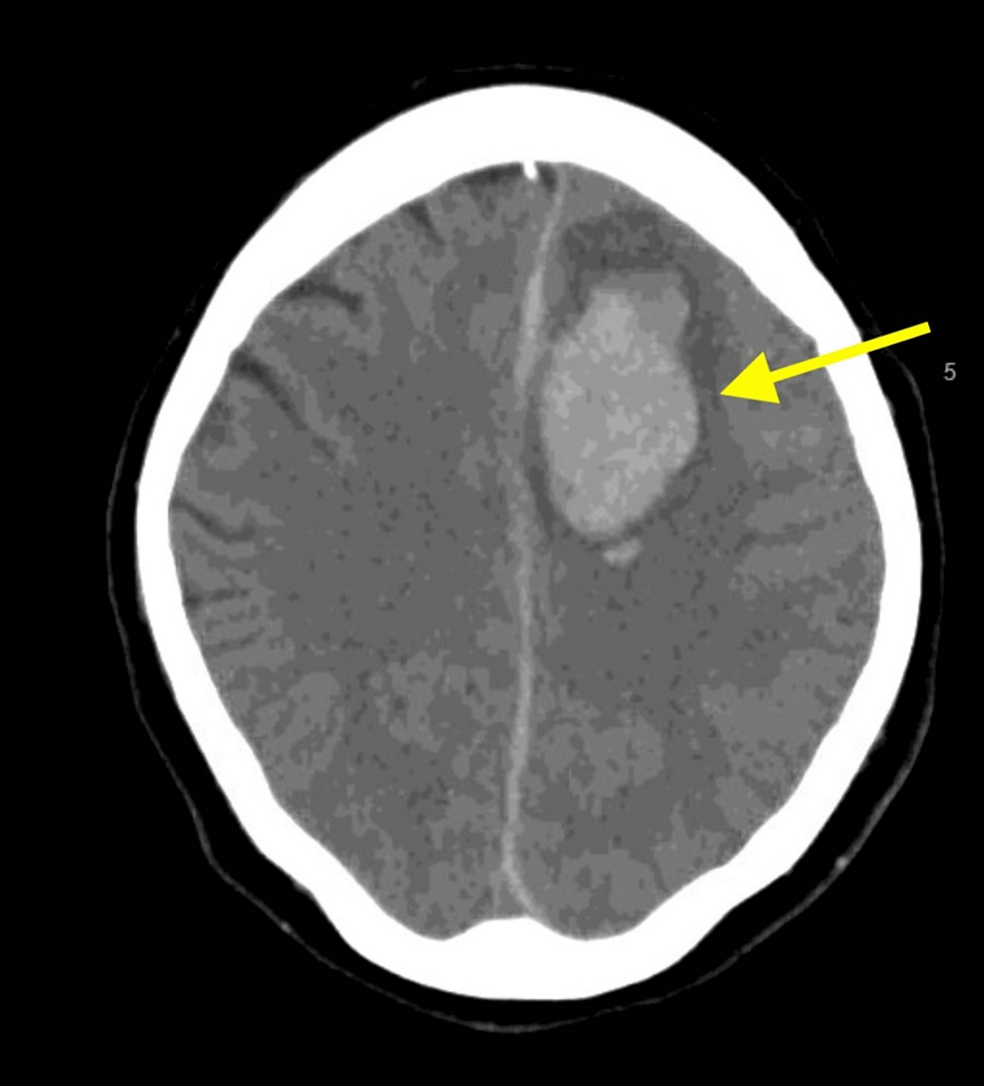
CT head without contrast after mental status change. An axial view of the CT of the head without contrast demonstrates a 4 cm left frontal parenchymal hematoma (arrow) with rightward subfalcine herniation. CT, computed tomography
